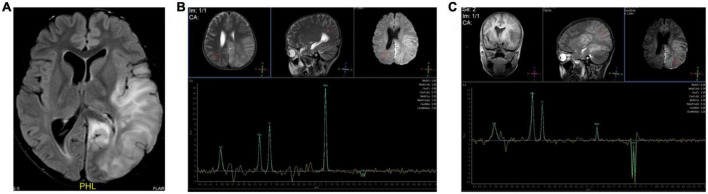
Elevated lactate doublet peaks on MRS. Case 12, A 12-year-old boy with MELAS. (A) Transverse FLAIR images showed cortical swelling in the left temporoparietal occipital lobe. (B,C) MRS showed elevated lactate doublet peaks in both acutely affected and unaffected regions; however, it was more obvious on the left.
